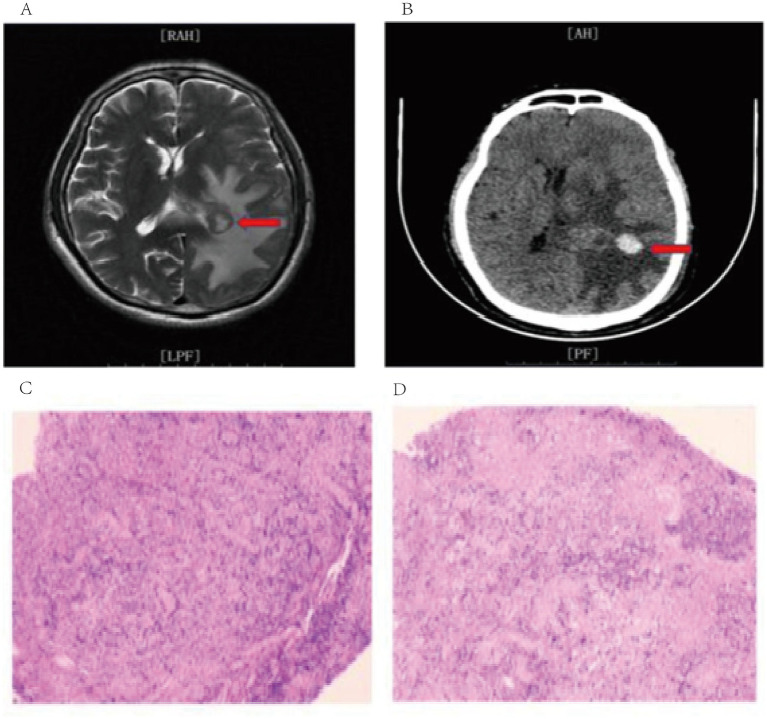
(A) Preoperative MRI imaging data; (B) Postoperative CT imaging data. (C, D) Postoperative pathology.
